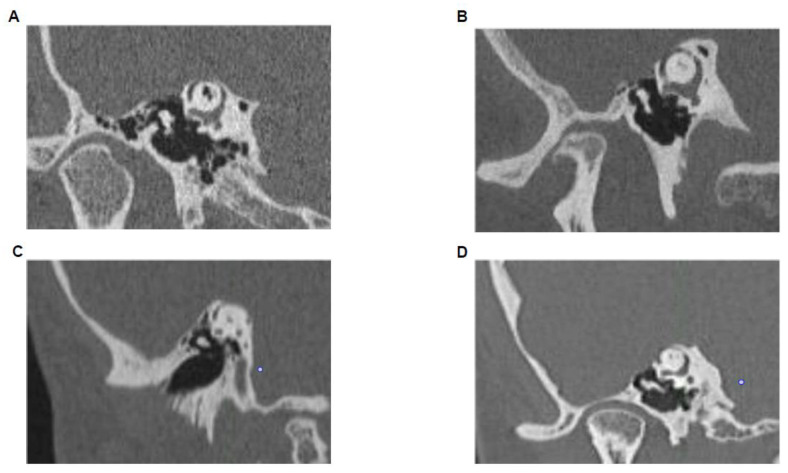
CT-Scan image representing each location. (A) Superior; (B) anterior; (C) posterior superior; (D) anterior superior.
